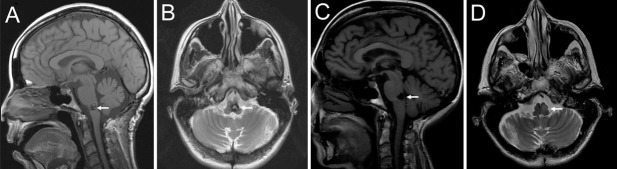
Brain magnetic resonance images of the pontomedullary junction cavernous malformation before and after resection. Preoperative sagittal T_1_- (A) and axial T_2_-weighted (B) images show heterogenous signal at the dorsal medulla (arrows) characteristic of a cavernous malformation with prior hemorrhage. Postoperative sagittal T_1_- (C) and axial T_2_-weighted (D) images show resection of the cavernous malformation and well-healed surgical site at the dorsal medulla (arrows).
